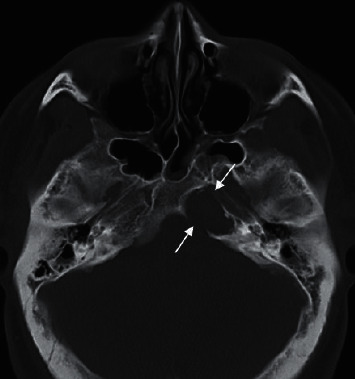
CT scan: left petrous apex smooth expansile lesion with internal soft tissue density and bone erosion.
